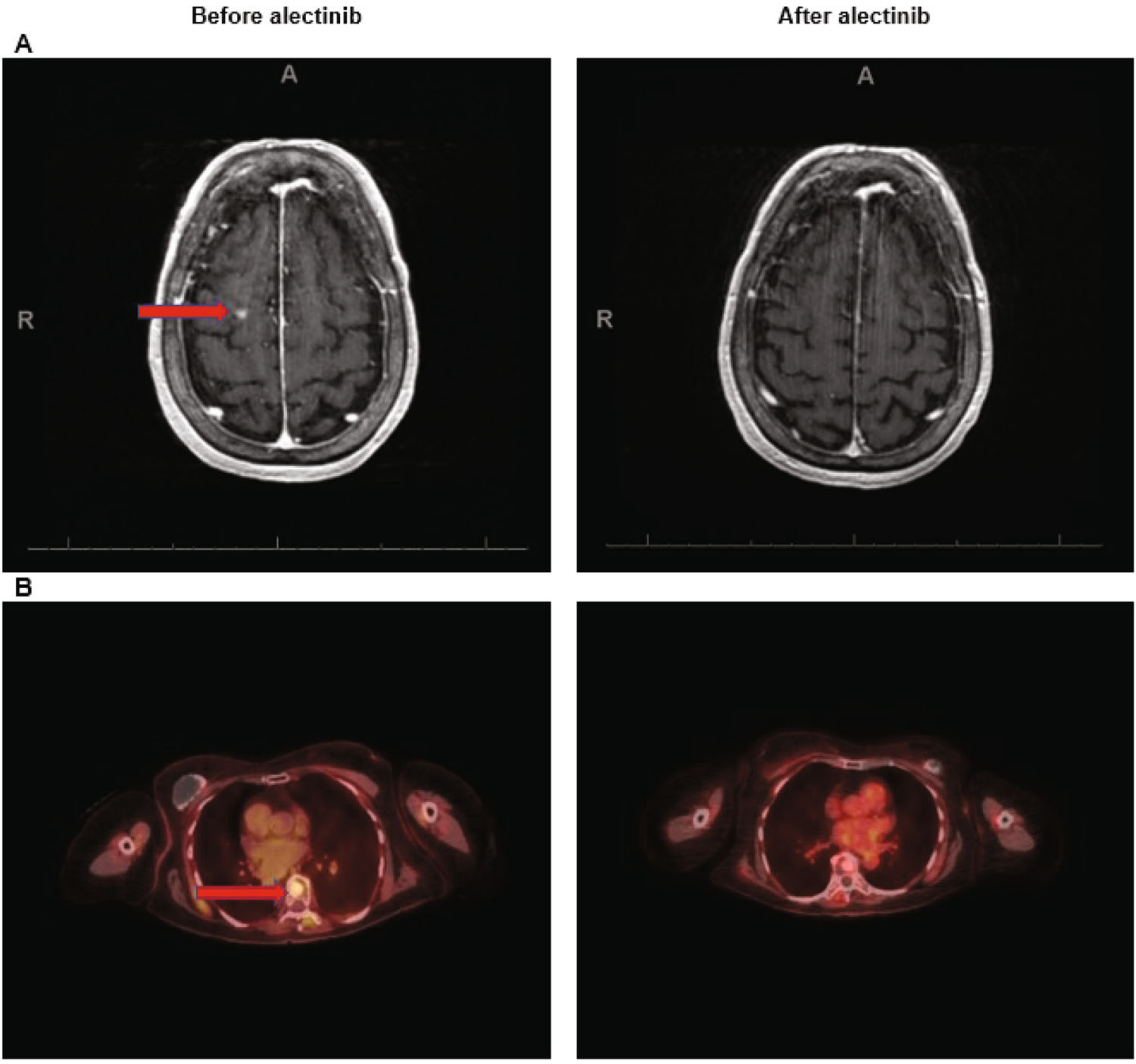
Case of 73-year-old woman with Erdheim Chester disease (non-Langerhans histiocytosis) and ALK-KIF4B fusion responds to alectinib. Figure showing response to alectinib in follow up scans taken 2 months after treatment initiation. A MRI brain showing resolution of the lesion in the right superior frontal gyrus (arrow). B PET/CT showing decreased FDG uptake in T8 sclerotic lesion. Red arrows show lesions in the before treatment scans.
